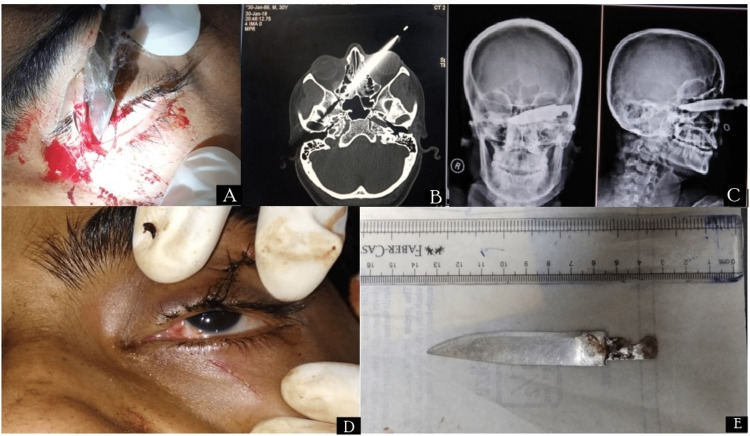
Case 1 (A) Image of stab injury with a knife in medial canthus; (B) non-contrast computed tomography (NCCT) of orbit showing foreign body (FB); (C) PA/lateral view X-ray of skull showing FB; (D) Image of patient post-FB removal; (E) FB shown with scale.
